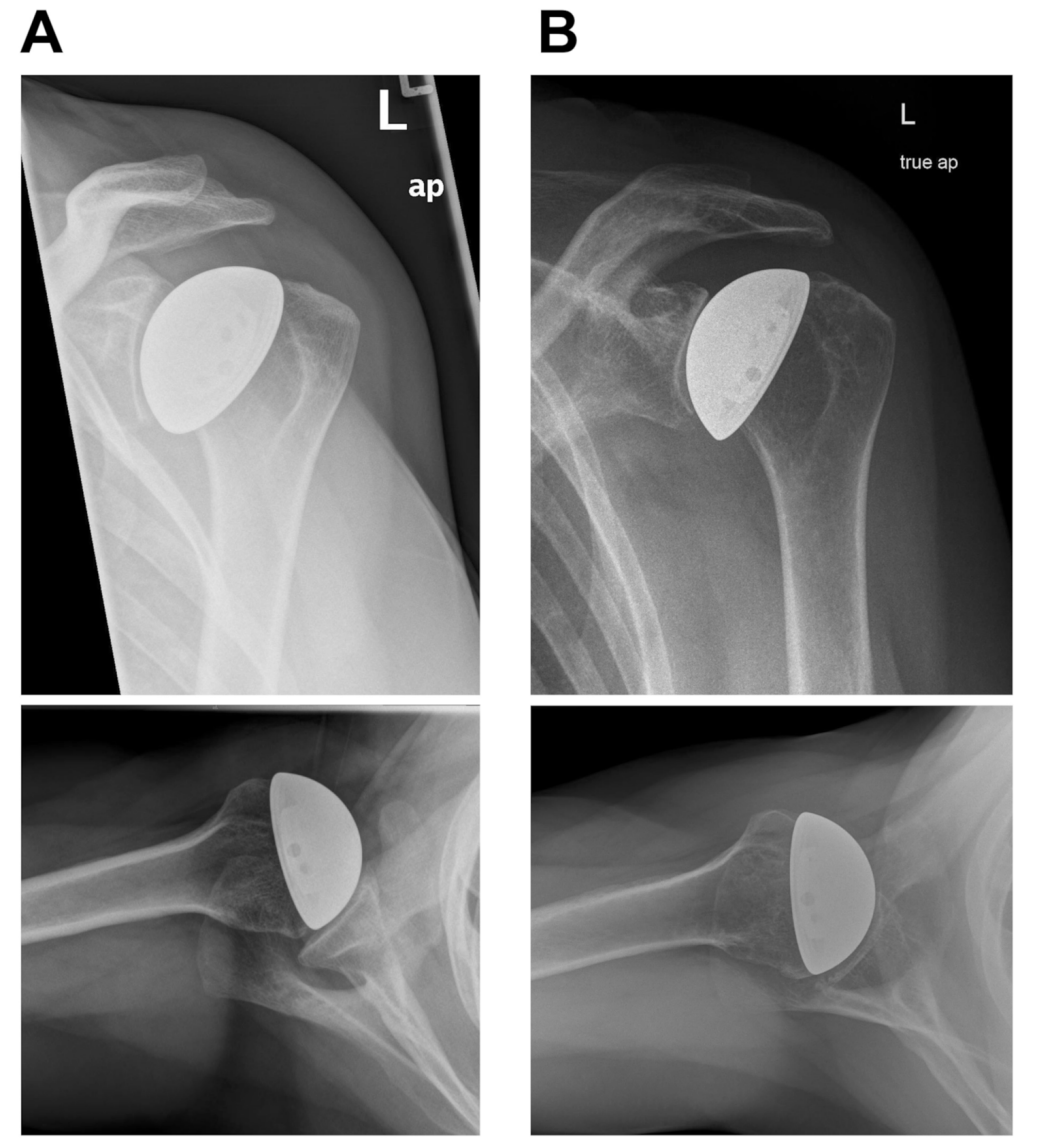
Radiological results of a postoperative course: (A) postoperative true a. p. (upper) and axial (lower) radiograph of a female patient operated at the age of 41 with diagnosed humeral head osteonecrosis Cruess stage 3 due to Hodgkin lymphoma treated with chemotherapy. (B) Follow-up radiograph true a. p. (upper) and axial (lower) at 17 years postoperatively showing mild glenoid erosion and a centered joint
